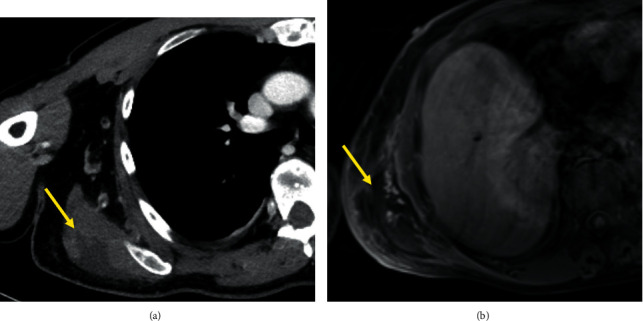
(a) Coronal images from a contrast CT of the thorax showing right latissimus dorsi muscle suggestive of deep tissue infection with possible muscle necrosis. (b) Coronal images from a contrast MRI of the thorax showing myonecrosis of the right latissimus dorsi muscle.
